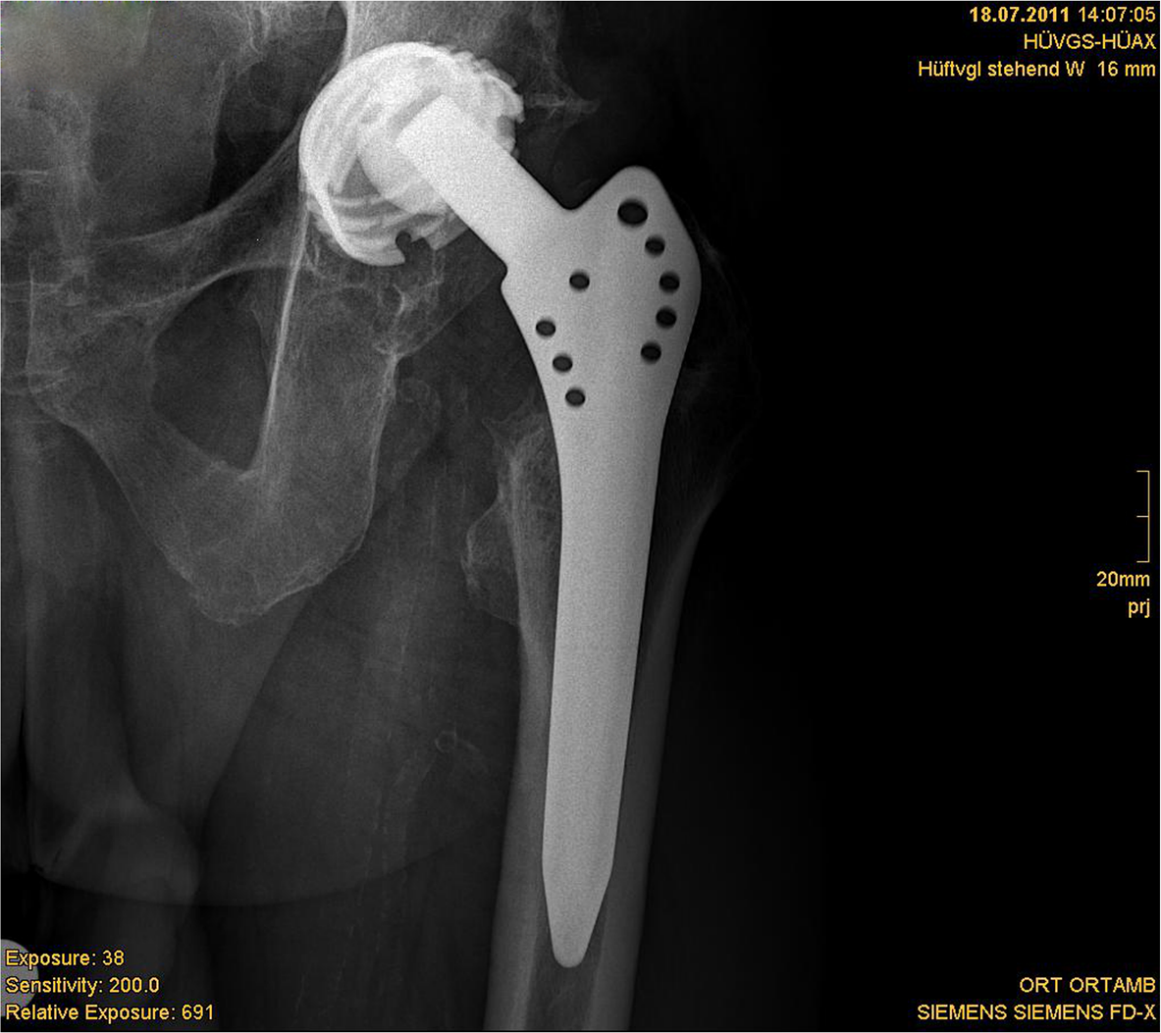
Radiograph showing totally worn-out liner after 25 years
